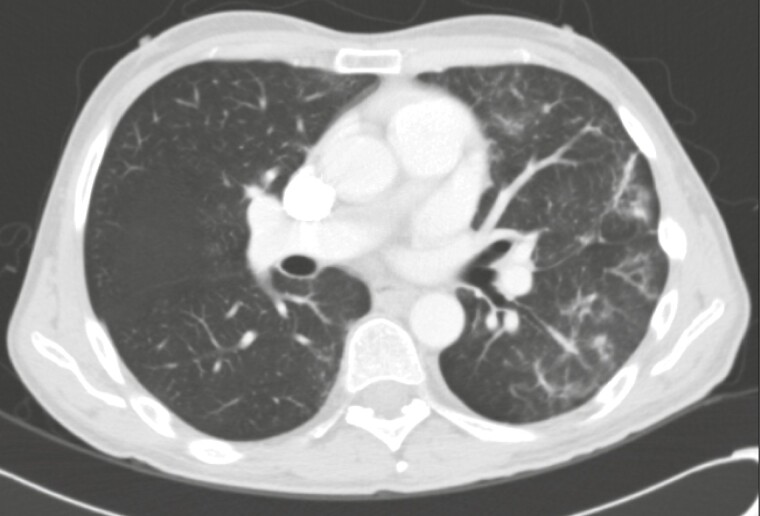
CT chest radiographic example of grade 1 pneumonitis. Unilateral multifocal subpleural ground-glass opacities.
